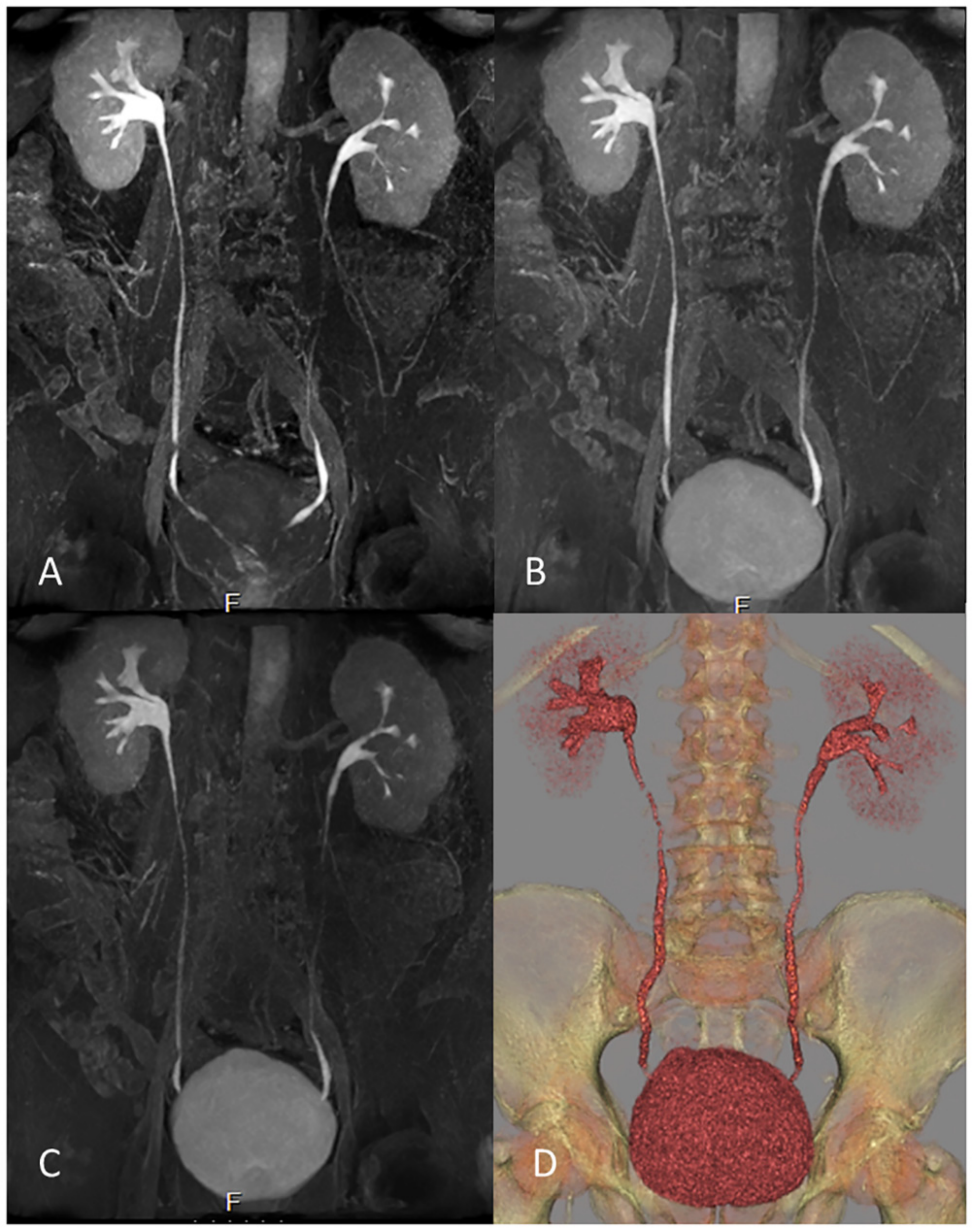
The value of MR imaging at different time intervals. MR urography maximum intensity projections at 5 min (A), 10 min (B) and 15 min intervals after the administration of contrast show no difference in visualization of the upper urinary tract (UUT) at MR-combined different time intervals. Different segments can be better visualized at different time intervals therefore improving the overall UUT visibility and provided comparable performance with CT urography (D, volume rendering reconstruction).
